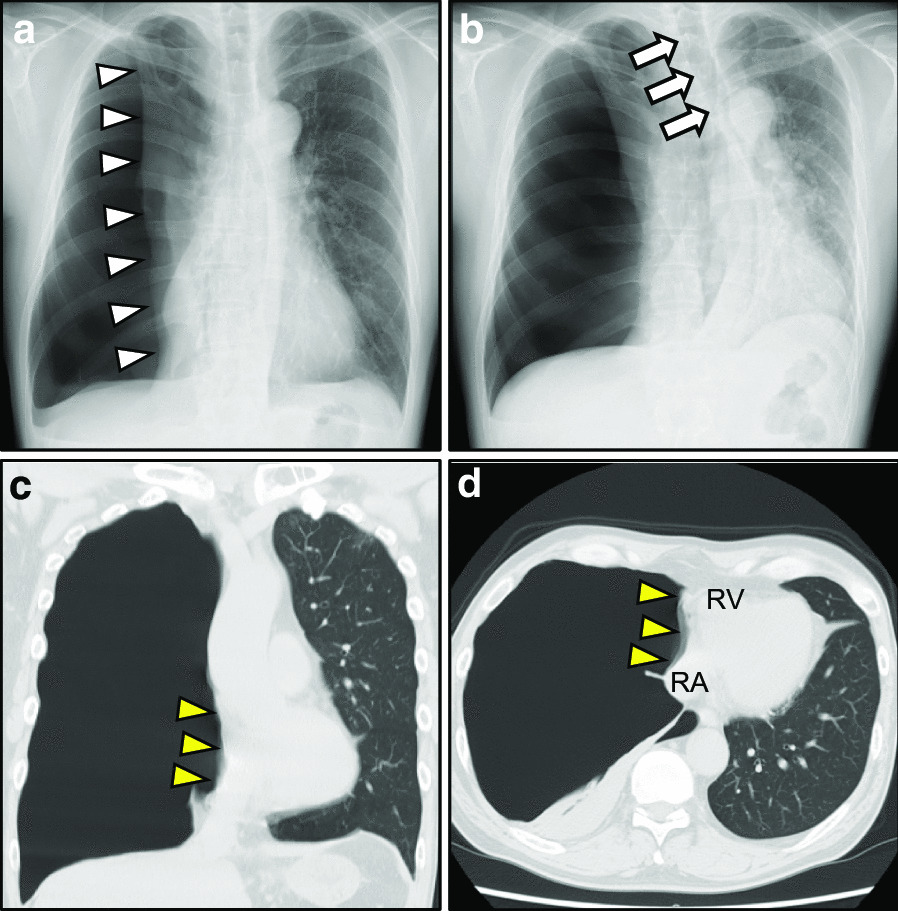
Initial chest radiographs and computed tomography scans (a, c, and d: during inspiration, b: during expiration). a Chest radiographs reveal a huge right-sided pneumothorax during inspiration. The absence of lung markings peripheral to the thin white visceral pleural line (white arrowheads) can be noted. b During expiration, increased intercostal space and a pronounced leftward mediastinal shift (arrows) are recognized. The hemidiaphragm on the right side remains quite depressed. c, d Chest computed tomography scans reveal a huge right-sided pneumothorax during inspiration. The extracardiac compression of the right atrial free wall result in a straightened cardiac border (yellow arrowheads). *RA* right atrium, *RV* right ventricle
